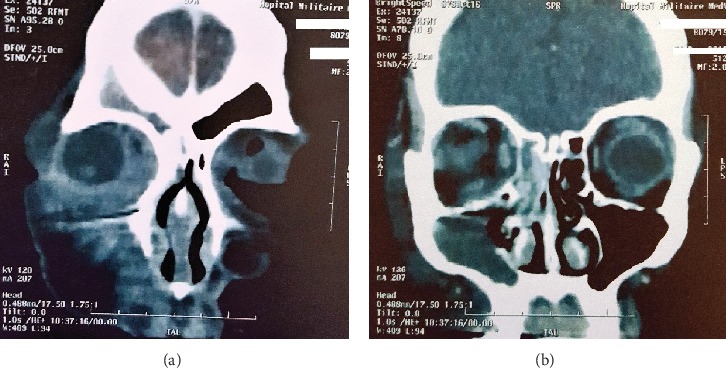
Orbitocerebral MRI scan in coronal sections showing extra and intraconical fat infiltration of the right orbit with filling of the right frontal sinus (a). Filling of the right maxillary and ethmoidal sinus (b).
